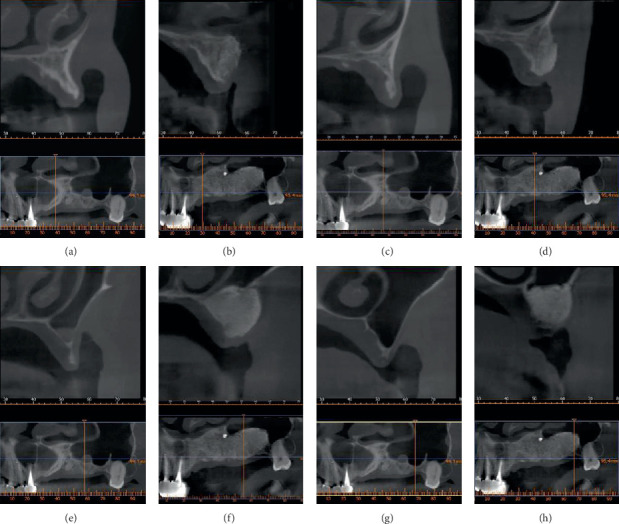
(a) Pre-op CBCT scan of the resorbed maxillary ridge on site 22. (b) Post-op CBCT scan of the newly formed bone on site 22. (c) Pre-op CBCT scan of the resorbed maxillary ridge on site 24. (d) Post-op CBCT scan of the newly formed bone on site 24. (e) Pre-op CBCT scan of the resorbed maxillary ridge on site 26. (f) Post-op CBCT scan of the newly formed bone on site 26. (g) Pre-op CBCT scan of the resorbed maxillary ridge on site 27. (h) Post-op CBCT scan of the newly formed bone on site 27.
